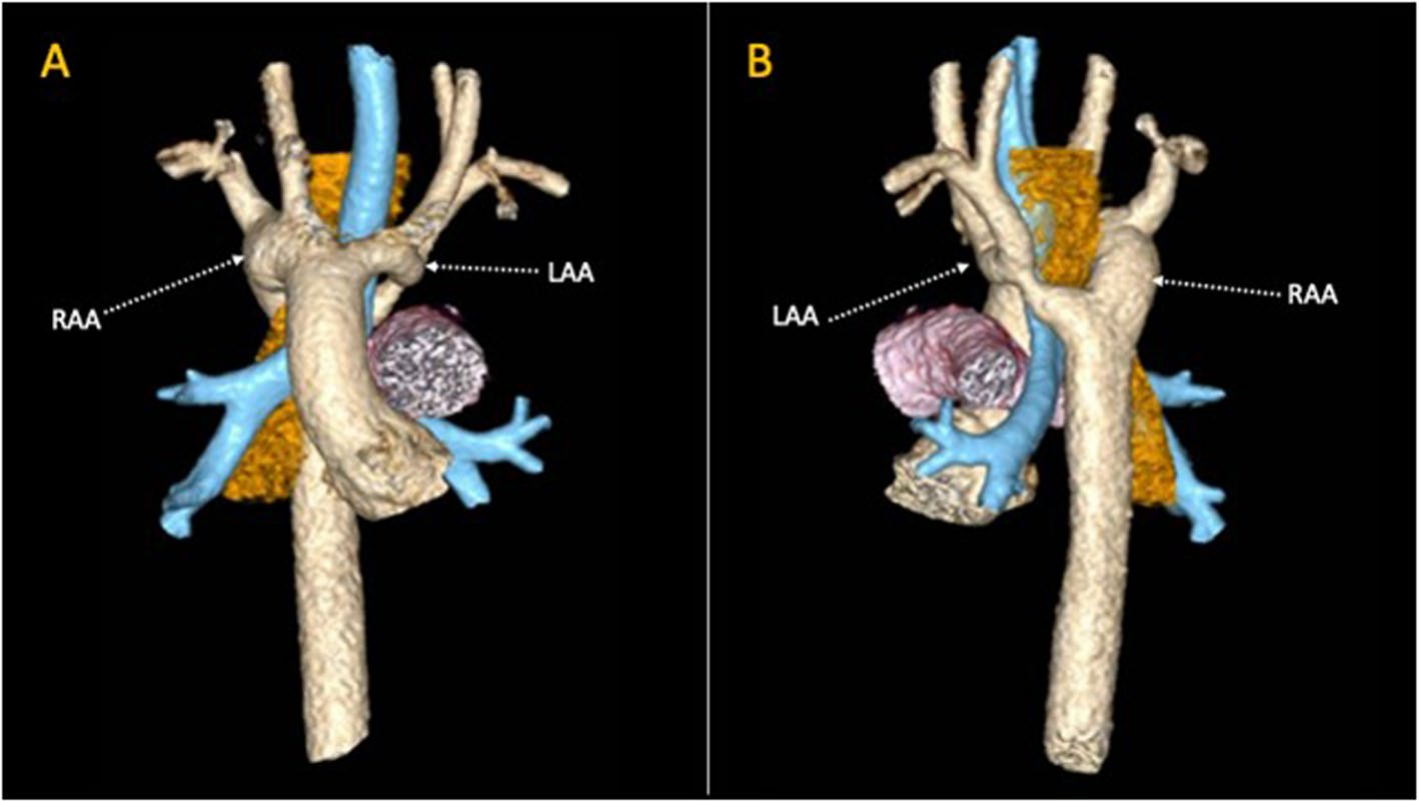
3D volume rendering reconstruction of a cardiac CT in patient with Double Aortic Arch, constituted by a dominant right aortic arch (RAA) and a diminutive Left Aortic Arch (LAA). The vascular structures together completely encircle the trachea (in blue) and the oesophagus (in orange). Pulmonary artery in violet. Panel A: front view. Panel B: back view
